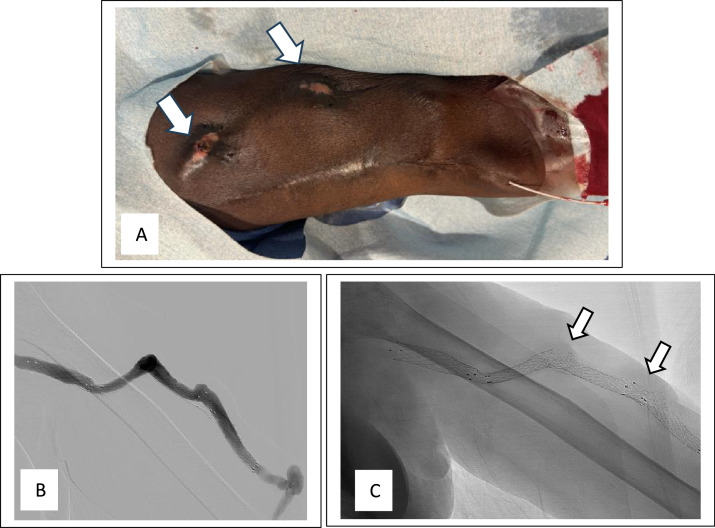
(A) skin ulcerated areas corresponding with the cannulation areas/fractured stents (arrows). (B) graft angiogram. (C) single shot X-ray showing fractured stents (arrows).
